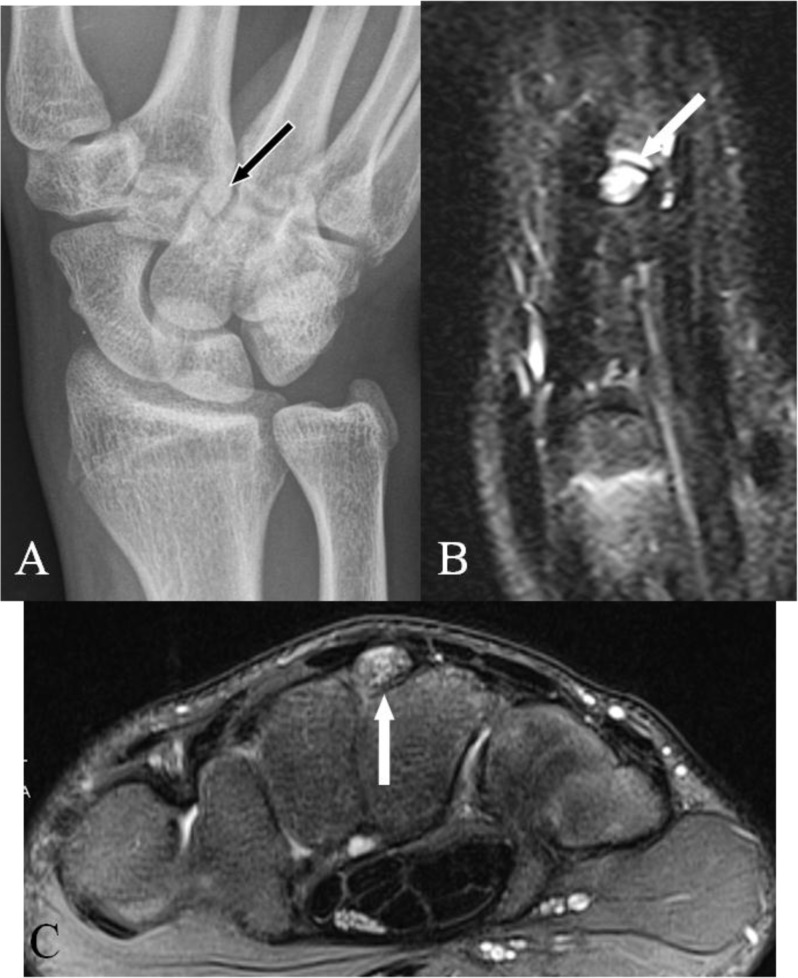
Acute painful CB in a tennis player. (A) Plain radiography PA view, (B) Coronal SE T2-WI FS and (C) Axial SE PD-WI FS. (A) Presence of an os styloideum (arrow). (B–C) Extensive bone marrow edema of the os styloideum (arrows), extending to the base of the third MC (B) (arrow).
